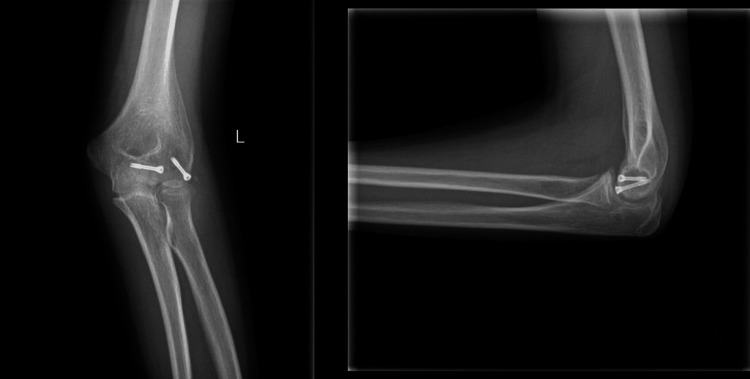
Postoperative anteroposterior and lateral radiograph on the last follow-up, six months postoperatively.
